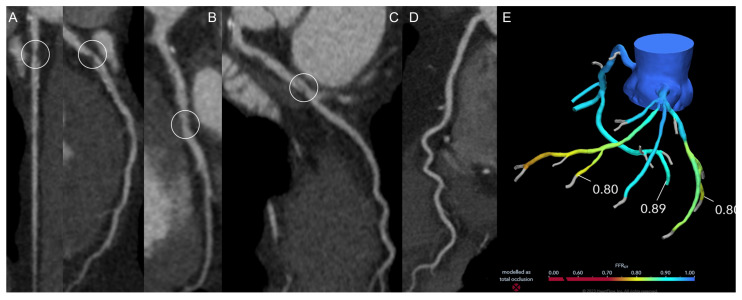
A 54-year-old male, with hypertension and dyslipidemia, presented for atypical chest pain onset (retrosternal dull chest pain with onset not reproducible at a definite threshold). CCT demonstrated moderate disease at proximal left anterior descending artery (white circles; (A)) and left circumflex artery (white circles; (B,C)). The right coronary artery had only minimal disease (D). FFR-CT was negative (E) and the patient was treated conservatively.
